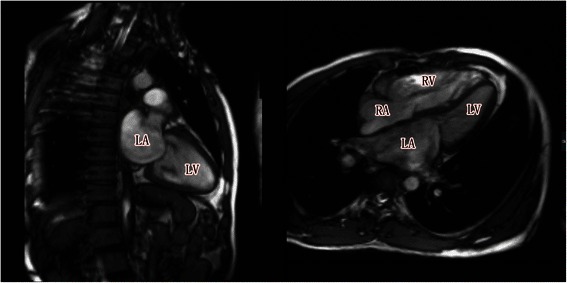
Magnetic resonance image showing thickening and opening restriction of the mitral valve.
